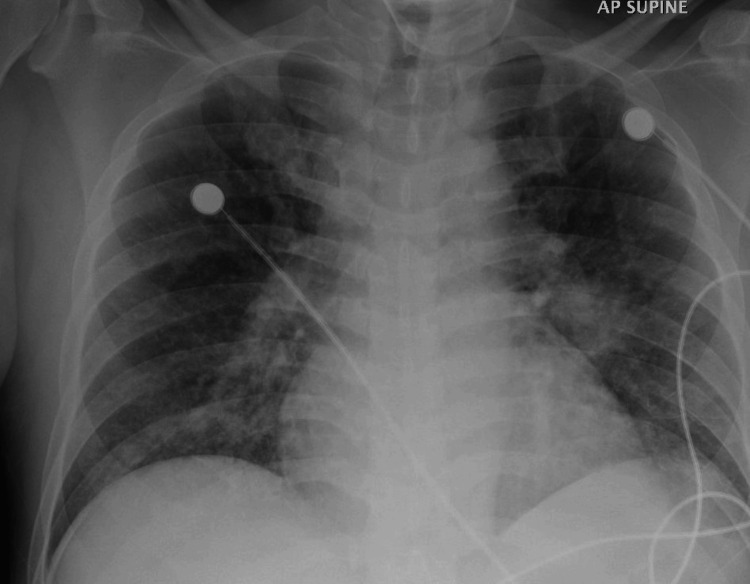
Chest X-ray at presentation
